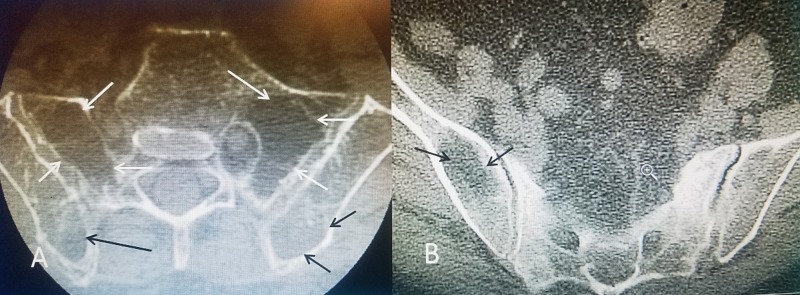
Axial CT showing sacral and iliac osteoporotic insufficiency defects A: CT scan of the sacrum and iliac wings showing severe osteopenia with loss of trabecula in the sacrum (solid white arrows) and similar but less severe change in the posterior iliac crest (solid black arrows); B: Loss of bone density in the middle part of the iliac wing more inferiorly (solid black arrows) CT: computed tomography
